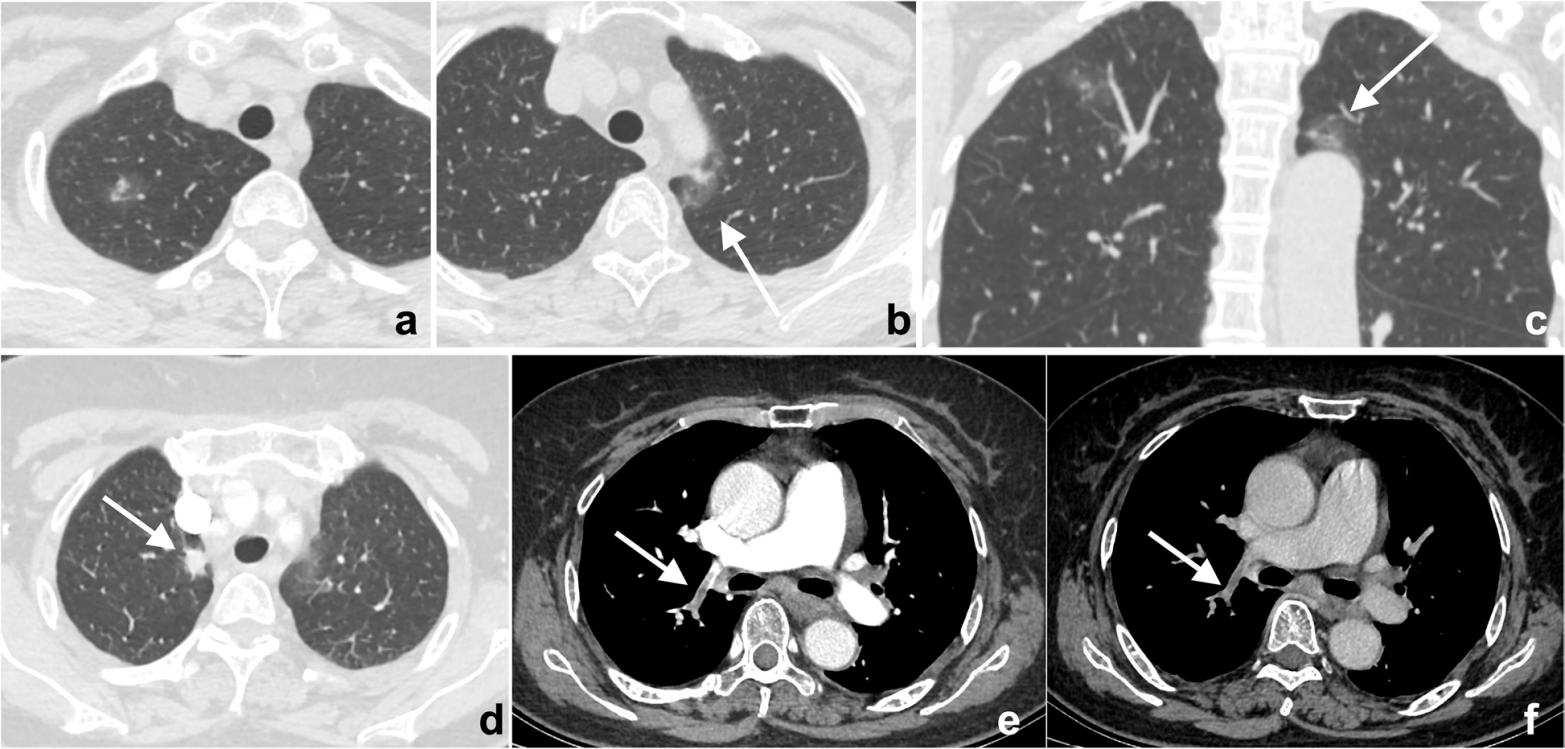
a–c Double primary lung adenocarcinoma in a 60-year-old woman. a Radiologists stopped searching for other lesions after detecting the subsolid nodule in the tip of the right upper lobe, missing another subsolid nodule (arrows) in the posterior apical segment of the left upper lobe, above the aortic arch and next to the mediastinum. Multiplanar reformation (MPR) demonstrated that the nodule (arrow) was above the aortic arch where there was a blind area commonly encountered in image reading. d–f A 72-year-old woman affected by COVID-19. The enhanced CT presented a description of the nodule (arrows) in the right upper lobe along with the burr sign of lobes, thus prompting the consideration of lung cancer. However, the low-density filling defect in the right upper pulmonary artery, which was a sign of pulmonary artery embolism, was missed
